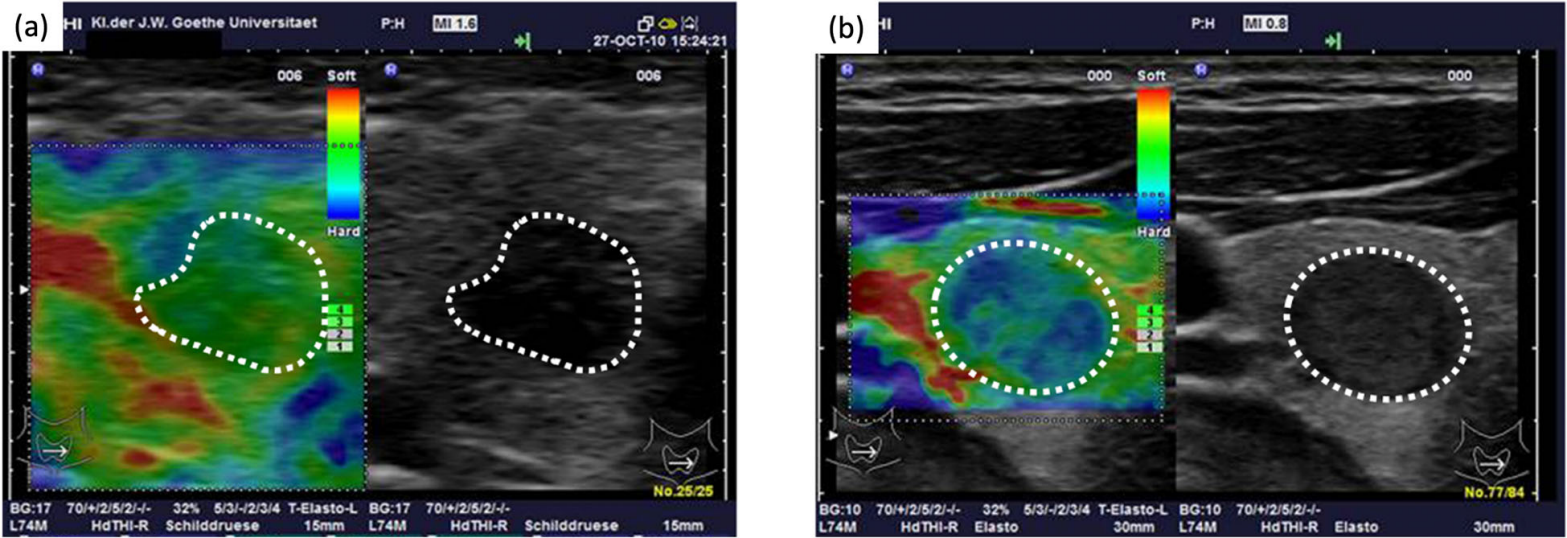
Ultrasound elastography. Elastography (colour-coded) and B-Mode images of a thyroid adenoma (a) and a papillary carcinoma (b) with the lesions encircled in white. The elastography images show clear differences and, thus, help characterize the carcinoma through reduced elasticity, as depicted by the blue regions, which correspond to a lower elasticity (image adapted from [150])
